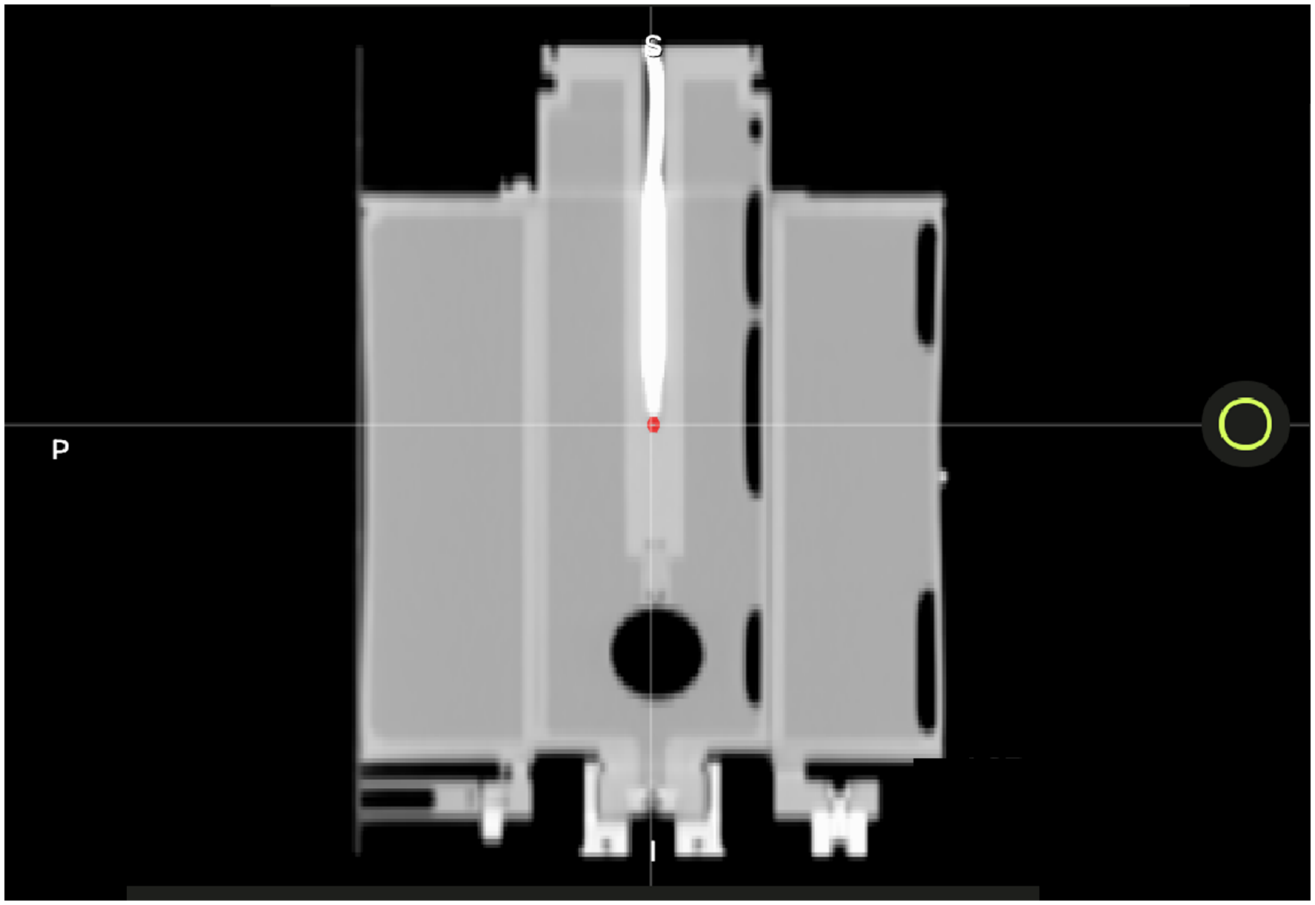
A CT image of the QUASAR with A26MR ionization chamber. The A26MR active volume is contoured in red.
